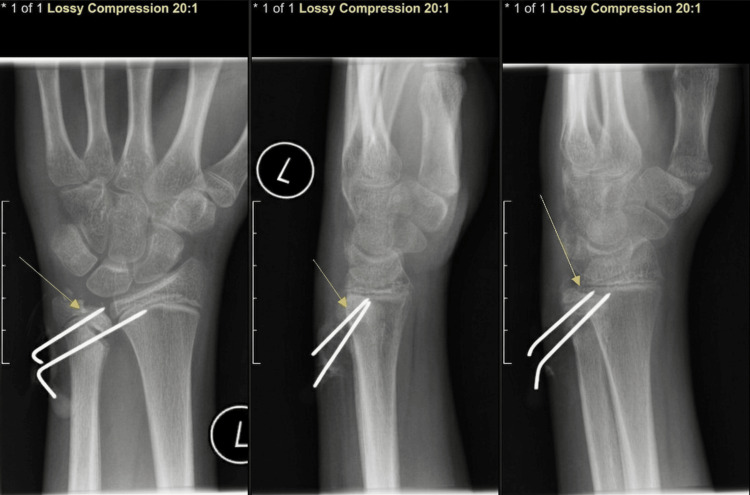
Radiographs at ~six weeks postoperative follow-up Radiographs of the left wrist anteroposterior/posteroanterior (AP/PA; left panel), lateral (middle panel), and oblique (right panel), demonstrating stable Kirschner wire position and maintained alignment (Day 45).
